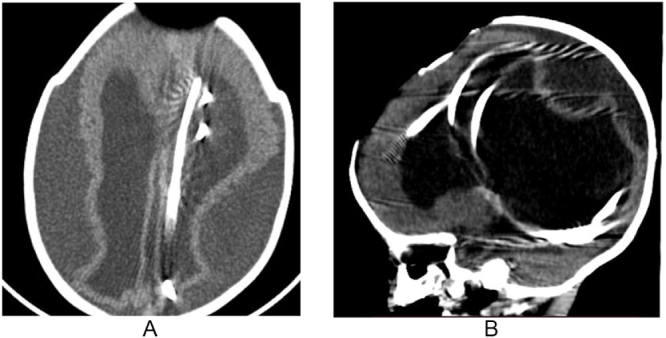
Axial CT (A) and sagittal (B) CT scan show migration of the shunt into the left lateral ventricle, dilation of the ventricular system, thin cortical mantle, and bilateral subdural collections.
